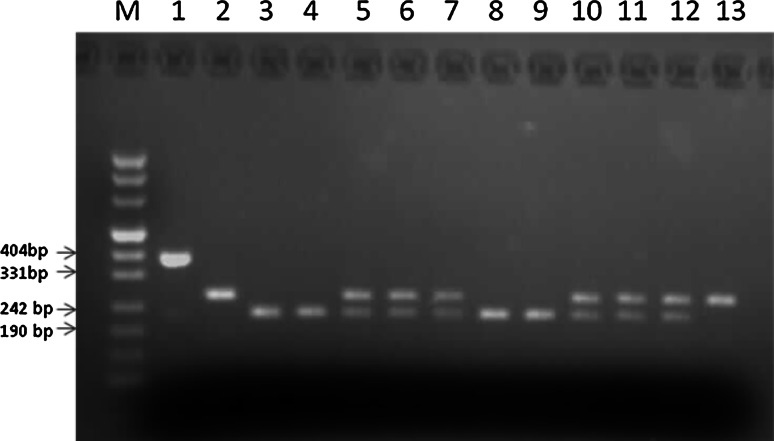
Identyfication of rs2476601 *PTPN22* gene polymorphism. *Lanes M* DNA length marker (Puc Mix Marker 8, MBI Fermentas), *1* uncleaved amplification product, *2*, *13* TT homozygotes, *3*, *4*, *8*, *9* CC homozygotes, *5*, *6*, *7*, *10*, *11*, *12* CT heterozygotes
